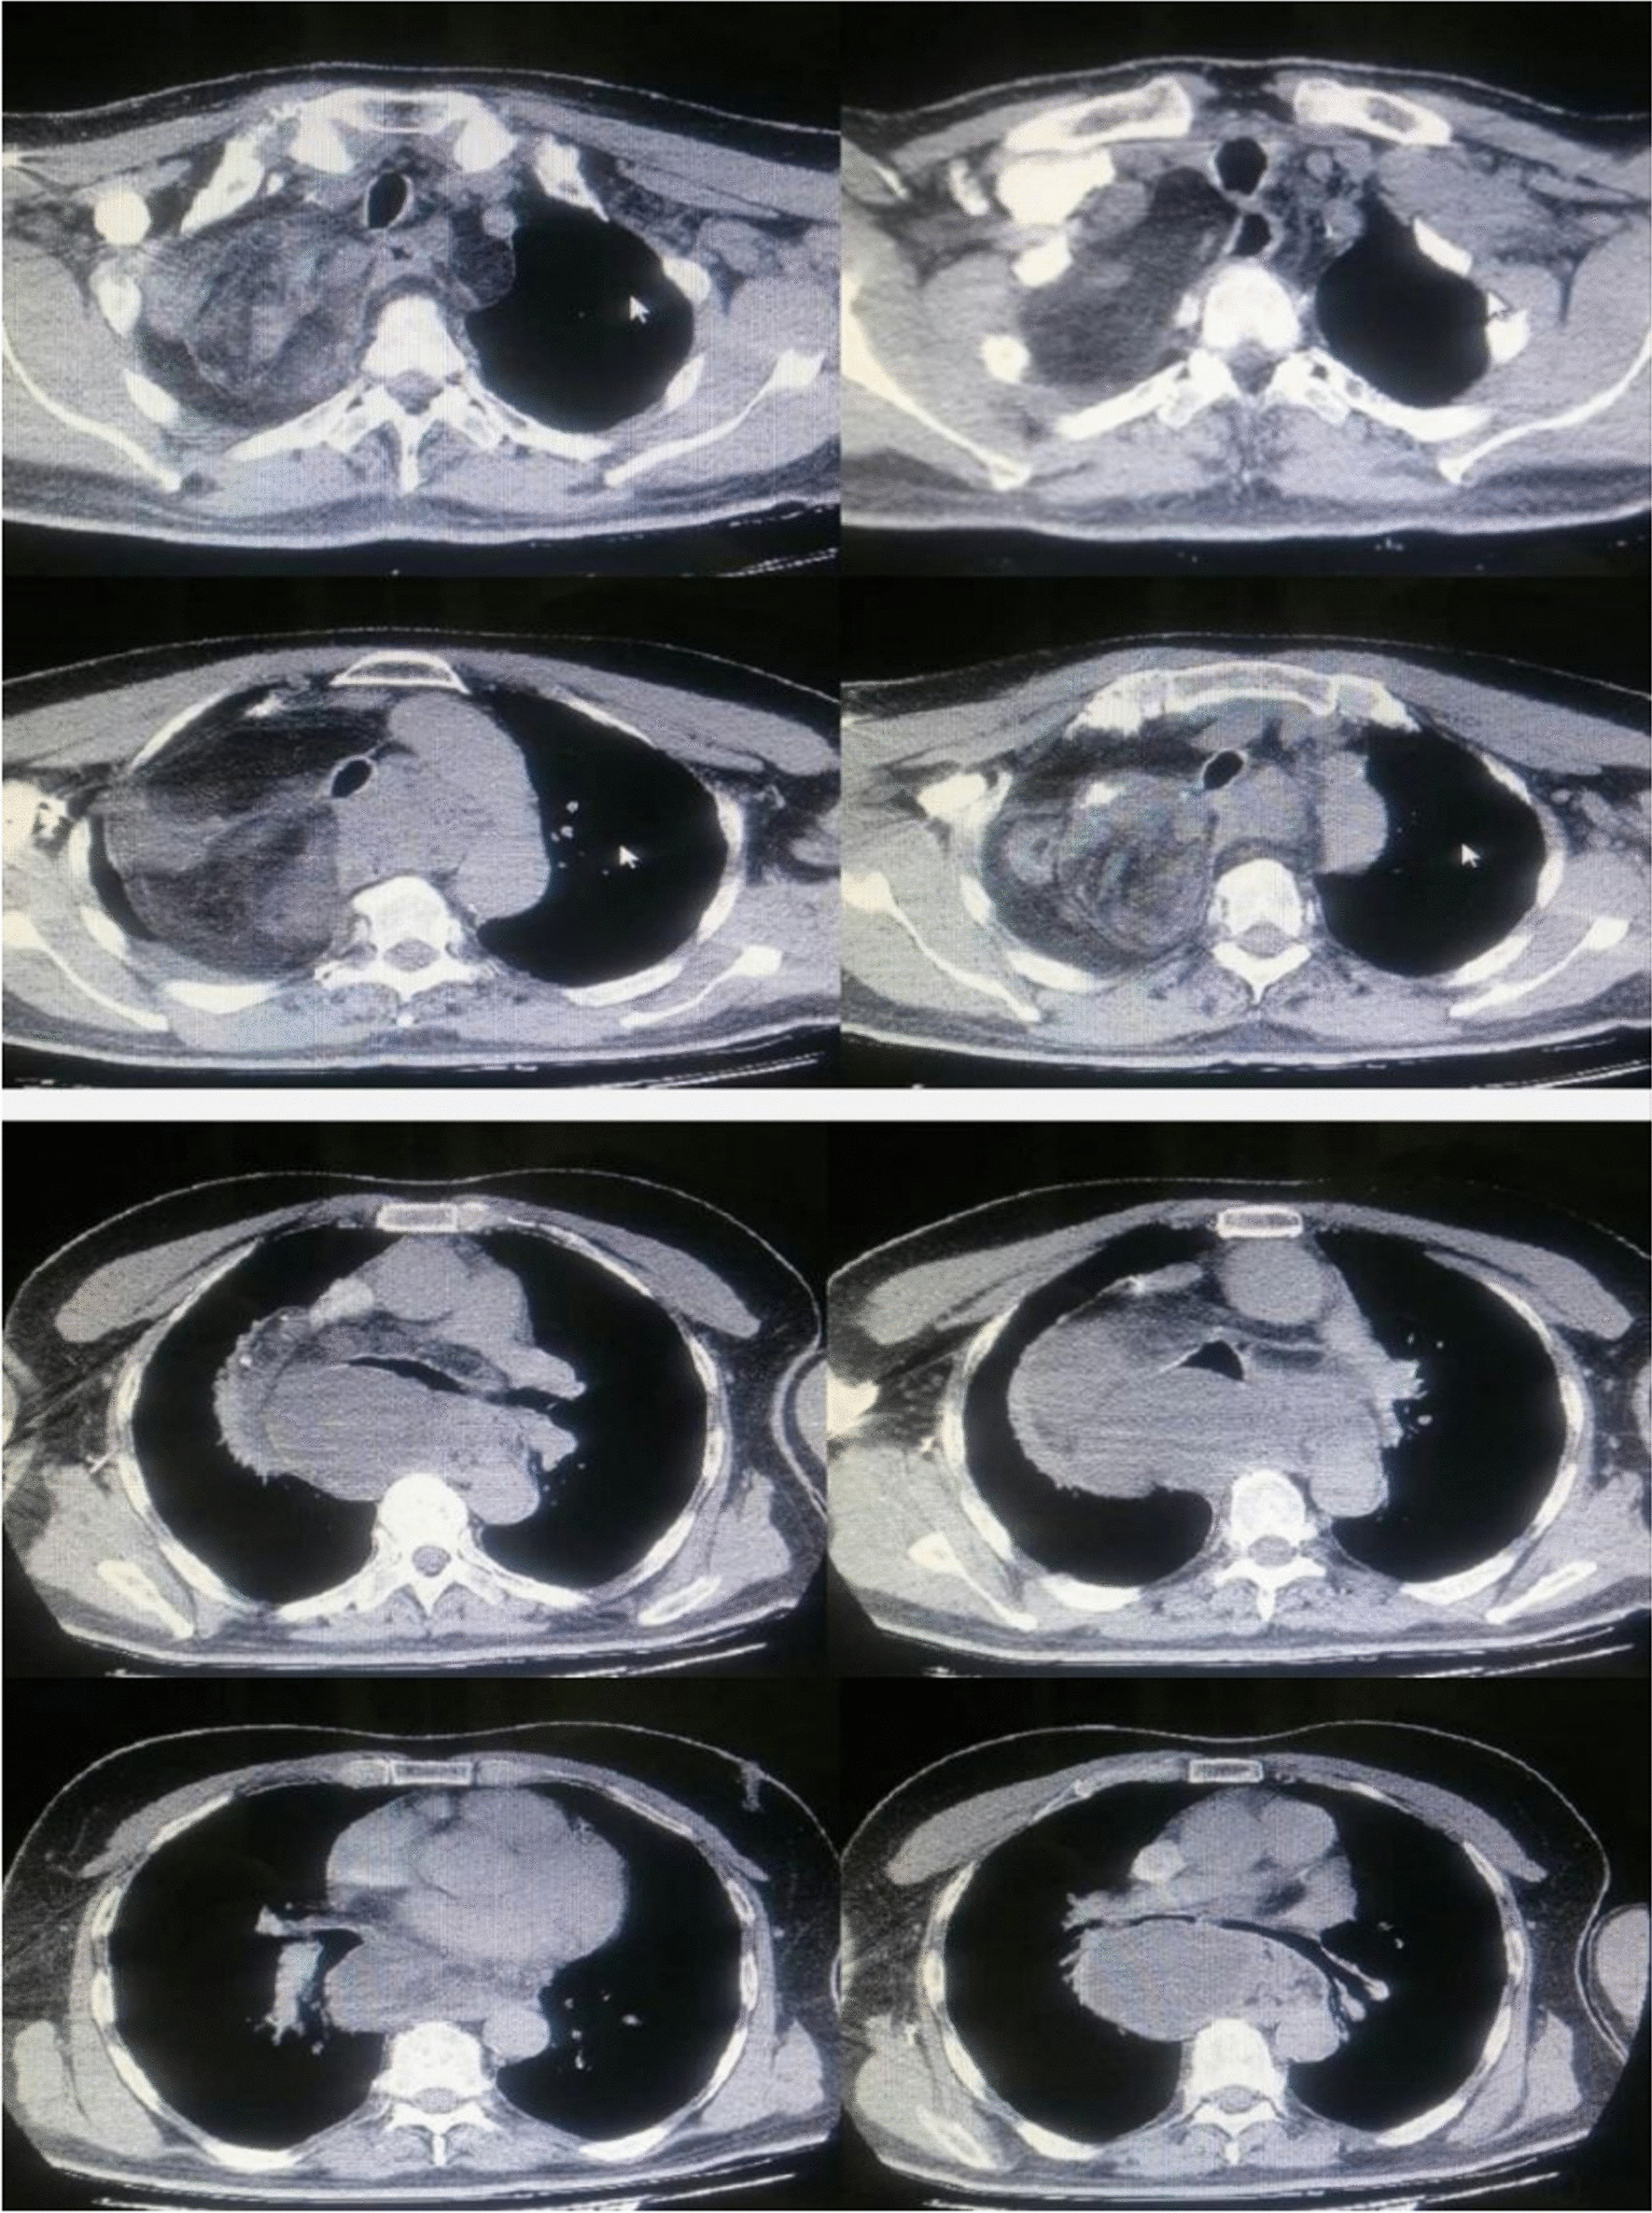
Presurgical Computed Tomography scan showing a mass with heterogeneous density involving the posterior mediastinum, spreading to the hilum, and upper lobe of the right lung
